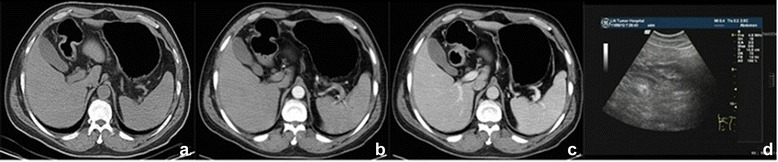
T2 gastric cancer in gastric antrum. (a-c) Enhanced CT scanning showed a clear gap surrounding the fat and smooth serosa. (d) The ultrasound imaging. Visible lesions invaded the muscle layer.
